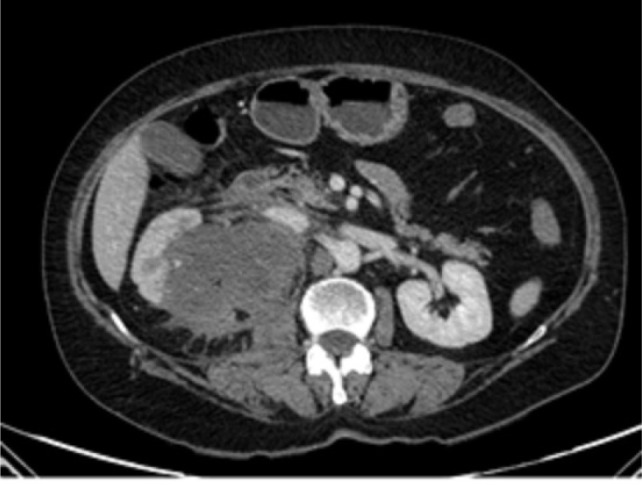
CT scan of the abdomen demonstrates the right renal mass.
